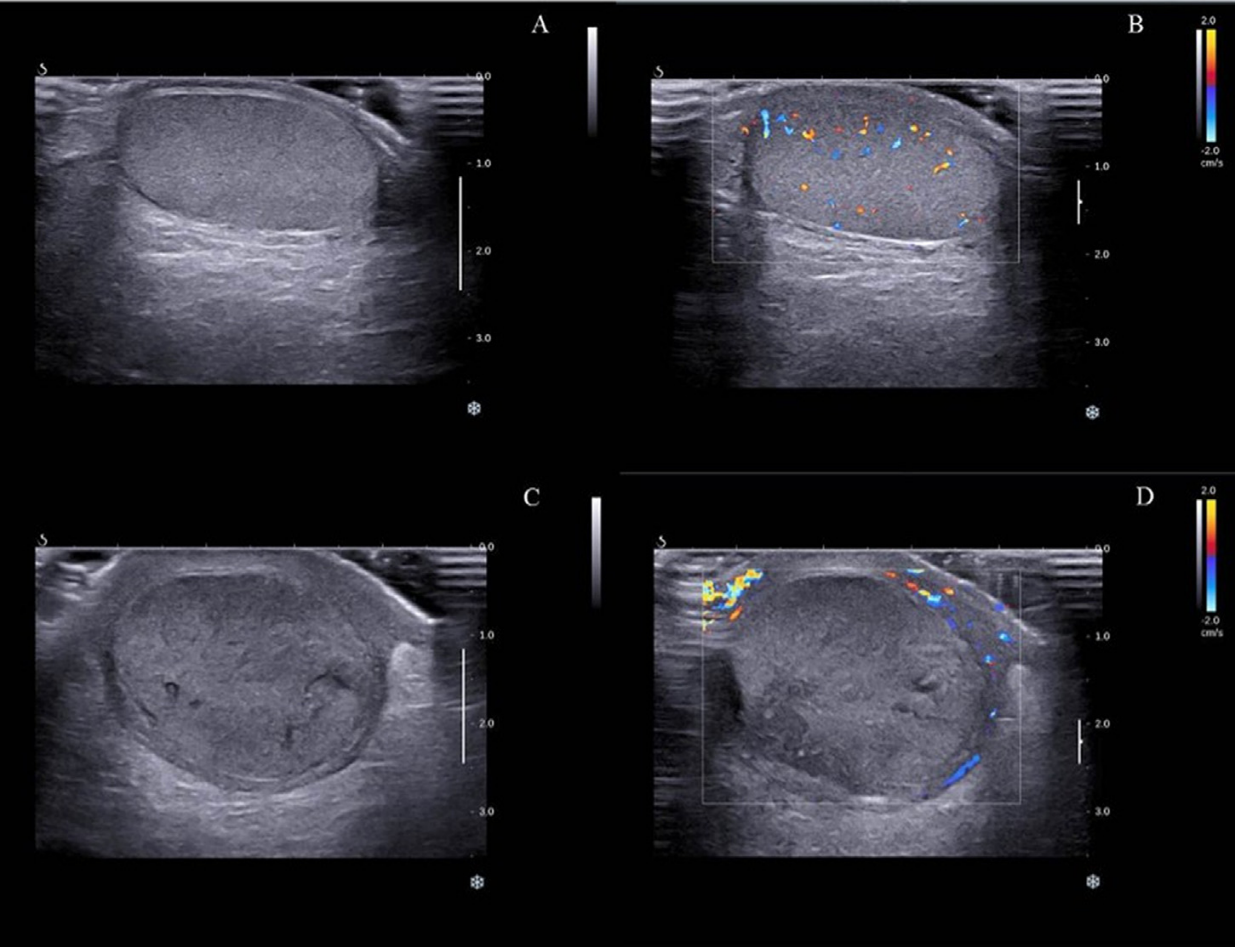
Gray-scale sonography and color Doppler sonography of twisted and normal testis. A: homogeneous testicular essence in the normal testicle. B: well blood flow signal inside in the normal testicle. C: swollen twisted testicle and heterogeneous echo inside. D: absent blood flow signal in the twisted testicle.
